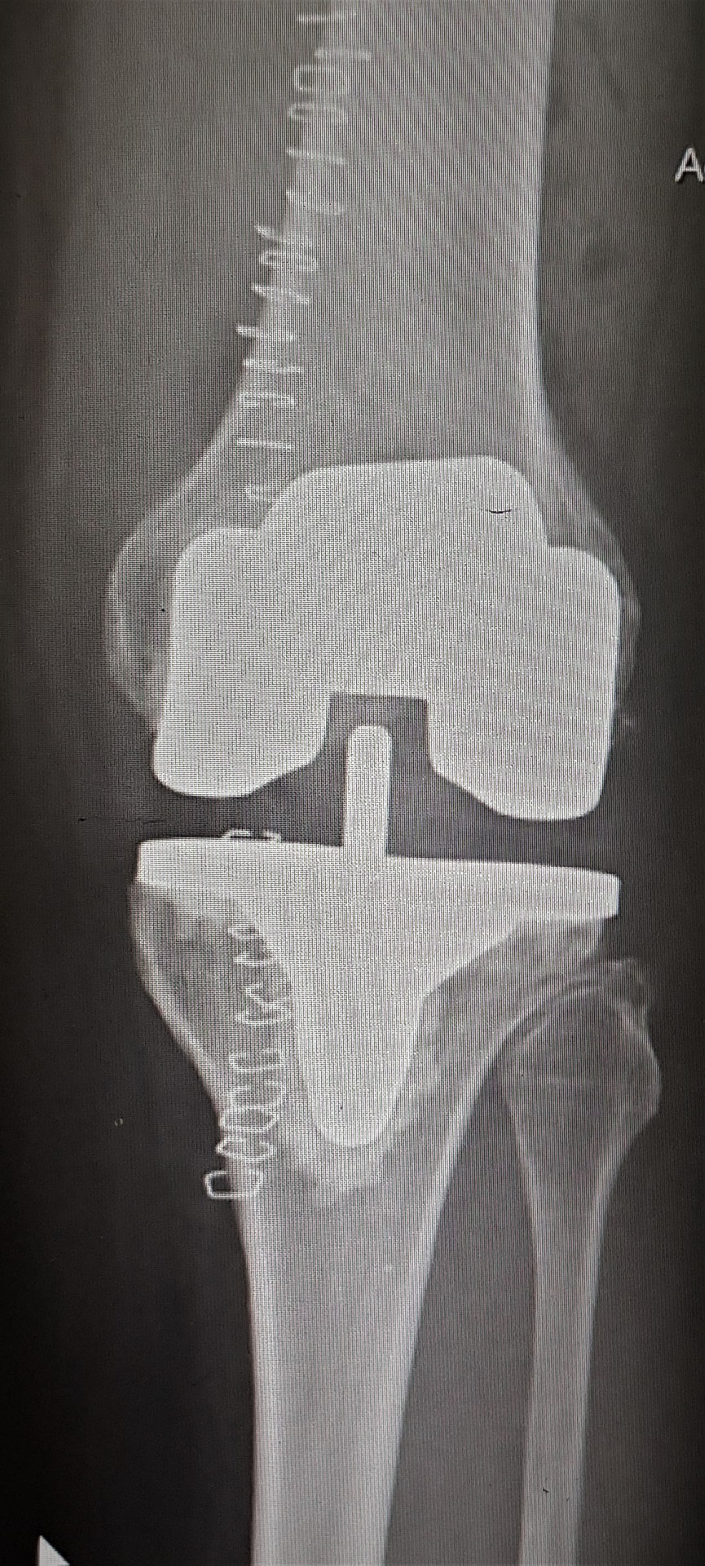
Radiograph of the left knee (anteroposterior view) with a stable implant in the immediate postoperative period
